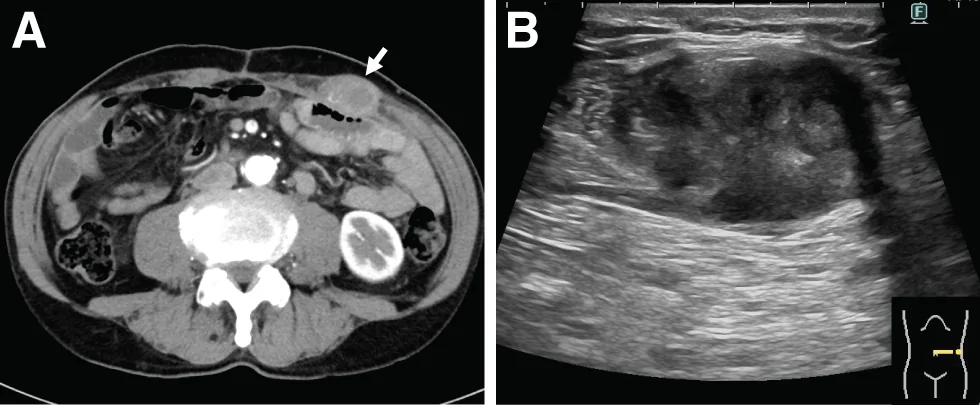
CT and ultrasonography findings of the PSR lesion. (A) Contrast-enhanced CT and (B) abdominal ultrasonography revealed a 3 × 4-cm irregular mass within the rectus abdominis muscle at the corresponding trocar site (white arrow). PSR, port-site recurrence
